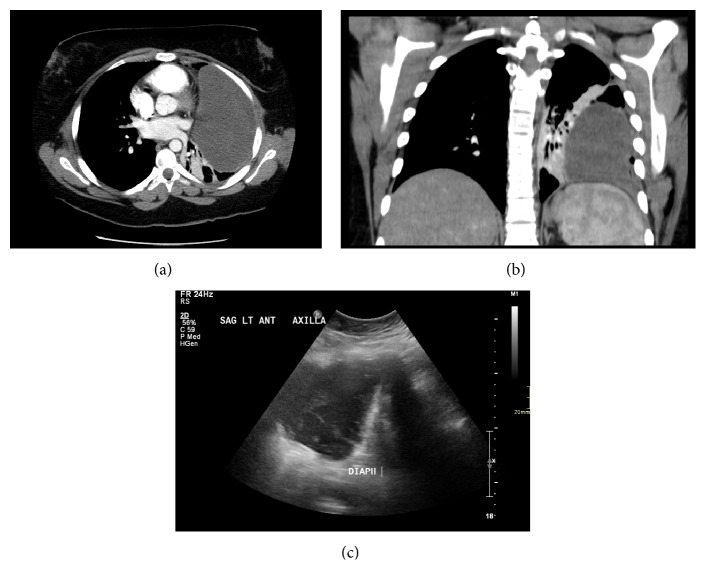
(a) Contrast enhanced axial image through upper thorax demonstrates a complex left pleural fluid collection with heterogeneous attenuation and internal septations. No vascularity was demonstrated to suggest and underlying mass. (b) Coronal image demonstrate partial collapse of the lower and upper lobes due to mass effect from the pleural collection. (c) Sonographic image through the left hemidiaphragm shows a mildly complex pleural fluid collection.
